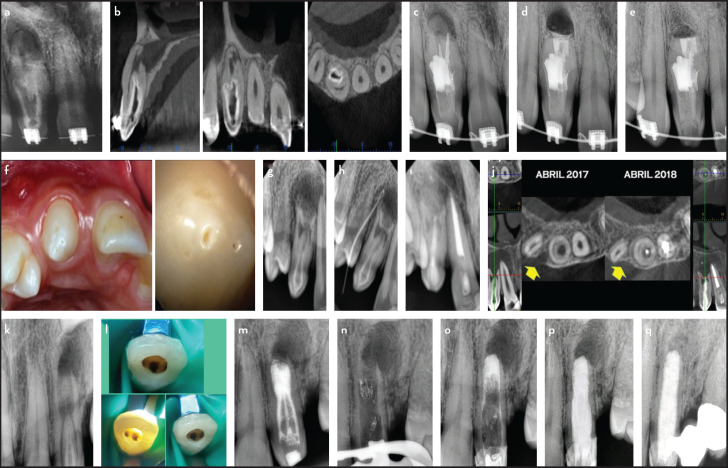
Type III DI cases and their management. 3 type III DI cases associated with large periapical lesions treated surgically (a-d), Non-surgical root canal treatment with warm vertical compaction (f-ı) and nonsurgical multi-appointment treatment with MTA apical plug and FRC post placement (k-p). Follow up after a year shows favorable healing (e, j, q)
